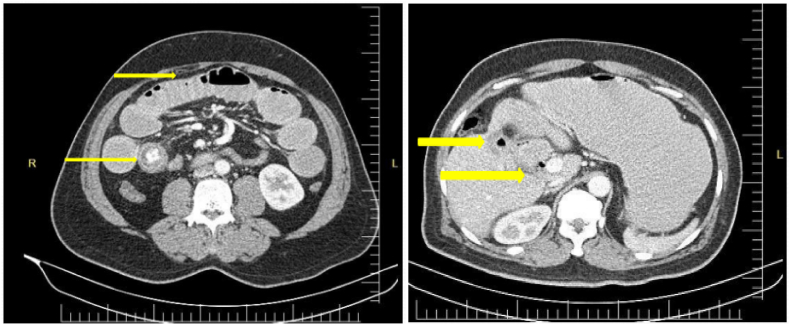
Computed Tomography scan revealed dilated bowel loops and concentric circles in the small intestine consistent with a gallstone ileus and decompressed GB and air in GB, verifying that a stone was released (yellow arrow). (For interpretation of the references to colour in this figure legend, the reader is referred to the Web version of this article.)
